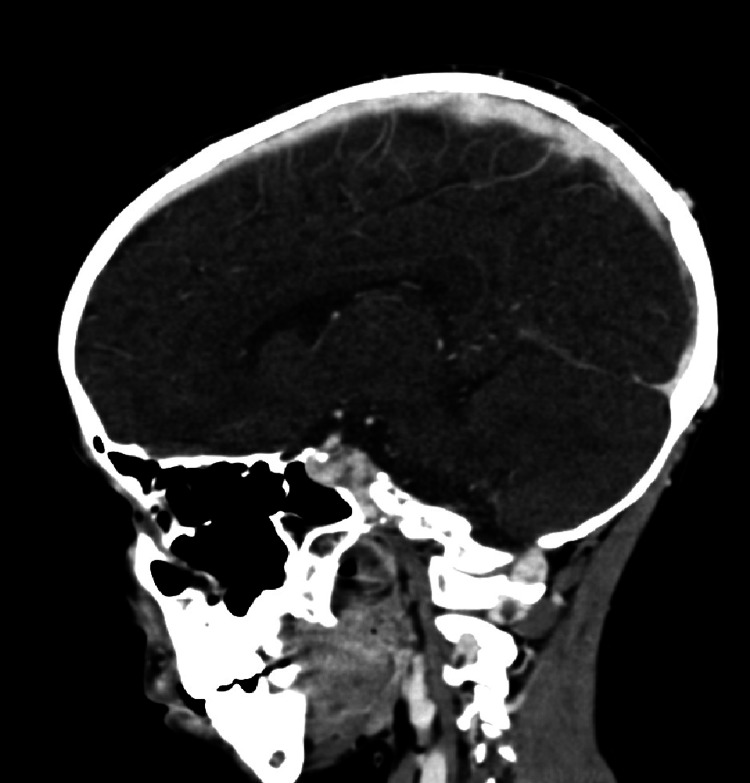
Sagittal CT image with contrast injection, performed two months after the initiation of anticoagulant therapy, showing absence of hypodensity within the superior sagittal sinus, indicative of complete resolution of the thrombosis.
